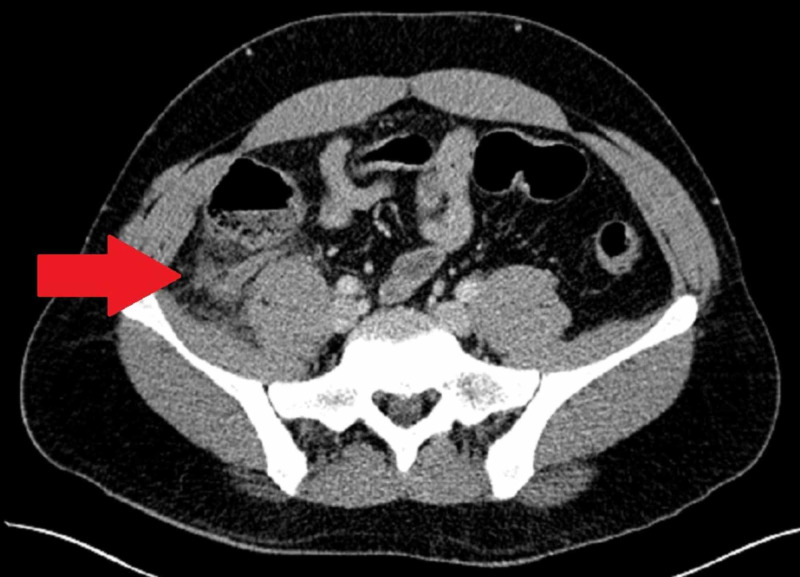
Perforated appendicitis on abdominal CT scan
